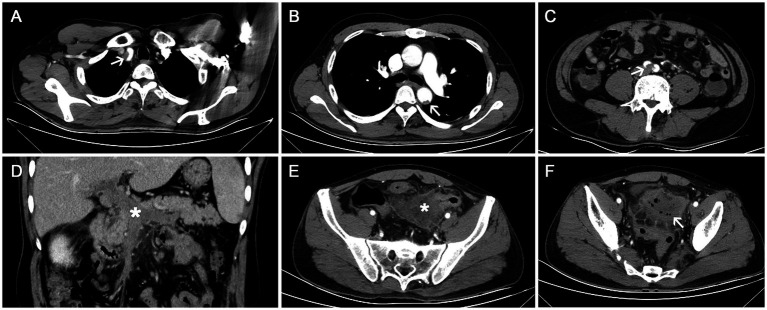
Contrast-enhanced computed tomography (CECT) scan. (A–C) Thrombosis in the brachiocephalic trunk, thoracic aorta, and bilateral iliac arteries (white arrows); (D) Thrombosis in the portal vein system (white star); (E,F) Wall thickening of the sigmoid colon with an abscess (white star) and extraluminal gas (white arrow), indicating perforation.
